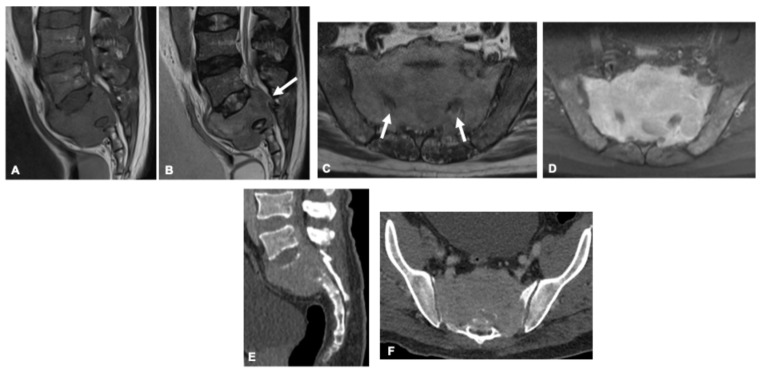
Plasmacytoma in a 61-year-old man. Sagittal and axial MR images (A–D) demonstrate a sacral osteolytic lesion with intermediate signal intensity on T1-weighted (A) and T2-weighted images (B,C), and diffuse, homogeneous post-contrast enhancement (D). Corresponding contrast-enhanced CT images (E,F) show extensive cortical destruction with extraosseous soft-tissue extension and marked narrowing of the spinal canal and sacral foramina, more evident on MRI (arrows).
